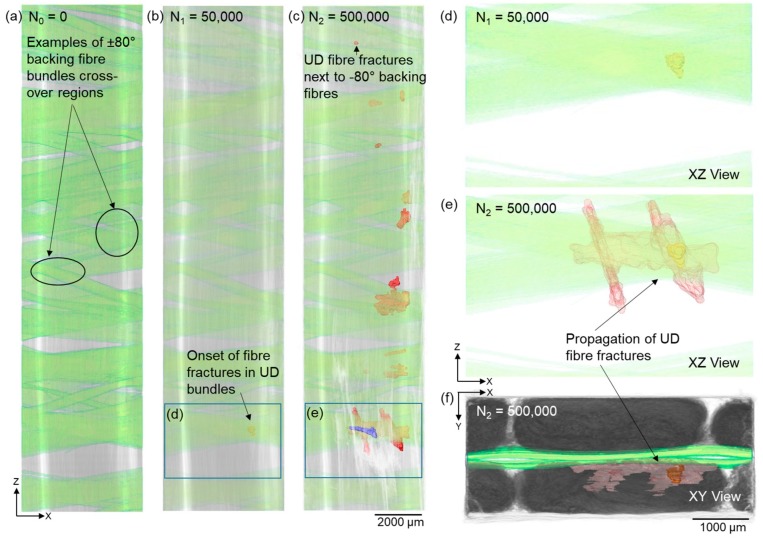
X-ray CT volume rendering of the backing fibre bundles (a) after 0 cycles showing the cross-over of the ±80° backing fibre bundles, as well as UD fibre fractures next to backing fibre bundles after (b) 50,000 and (c) 500,000 cycles, together with magnified views in (d,e) and in (f) the evolution and distribution of UD fibre fractures initiated from backing bundles after 500,000 cycles (refer to Figure 3 for explanations of colour coding). For 3D visualisation see the supplementary video.
